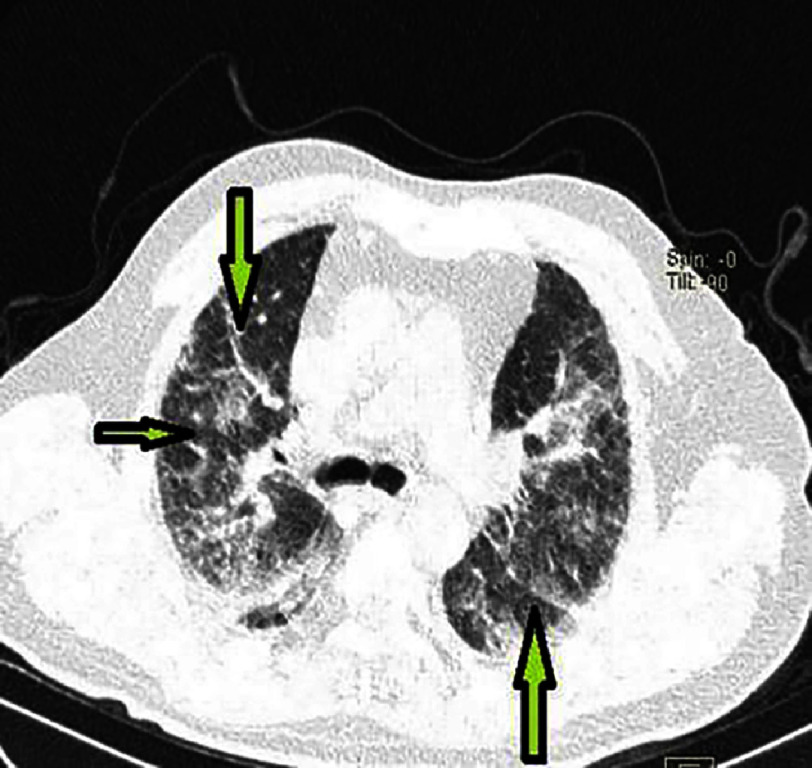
Chest CT demonstrating multiple bilateral ground-glass opacities and mild septal thickening on lung parenchyma view in patient #1.
